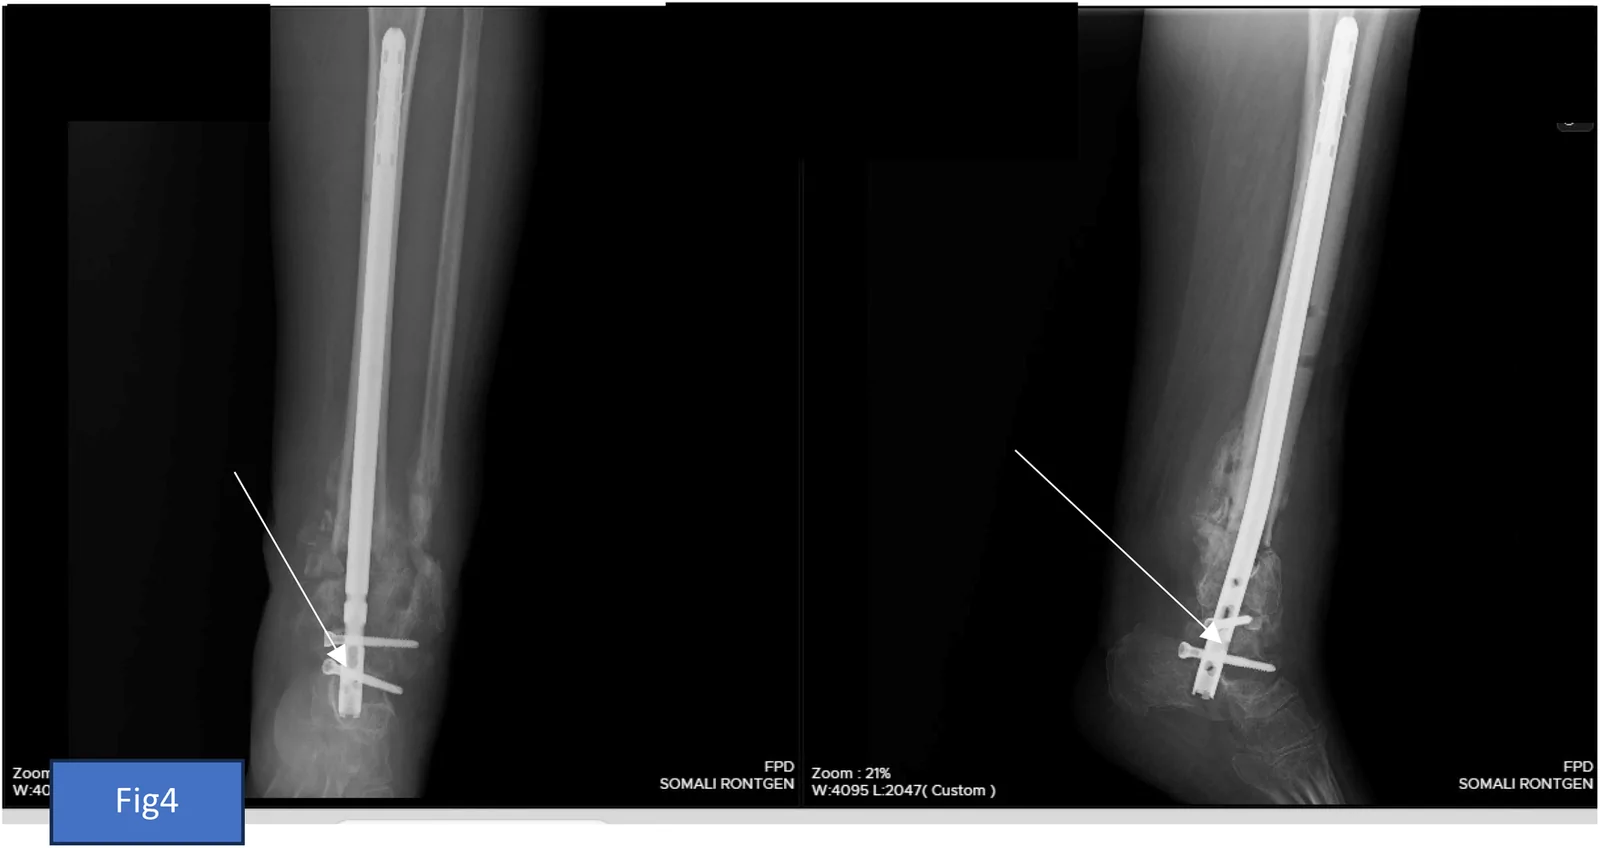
Definitive reconstruction with ankle arthrodesis. (A) Anteroposterior and lateral radiographs obtained 6 months after definitive ankle arthrodesis demonstrating intramedullary nail fixation with maintained alignment and progression toward fusion. The arrow indicates the ankle arthrodesis site.
